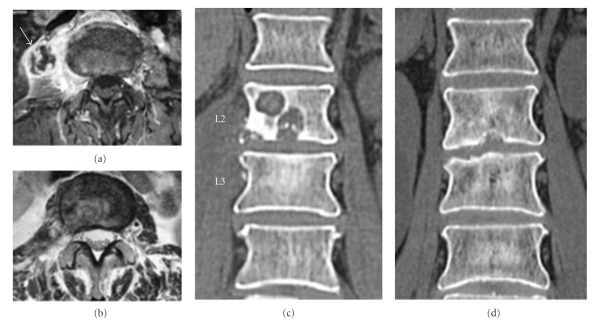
(a) Preoperative gadolinium imaging, axial image. Psoas abscess formation is visible (arrow), along with rim enhancement. (b) T2-weighted imaging at 3 months postoperatively, axial image. The iliopsoas abscess has disappeared. (c) Preoperative CT shows clear cavitations within the L2 vertebral body. (d) CT at 12 months postoperatively indicates that the cavity within the vertebral body has disappeared and has been remodeled.
